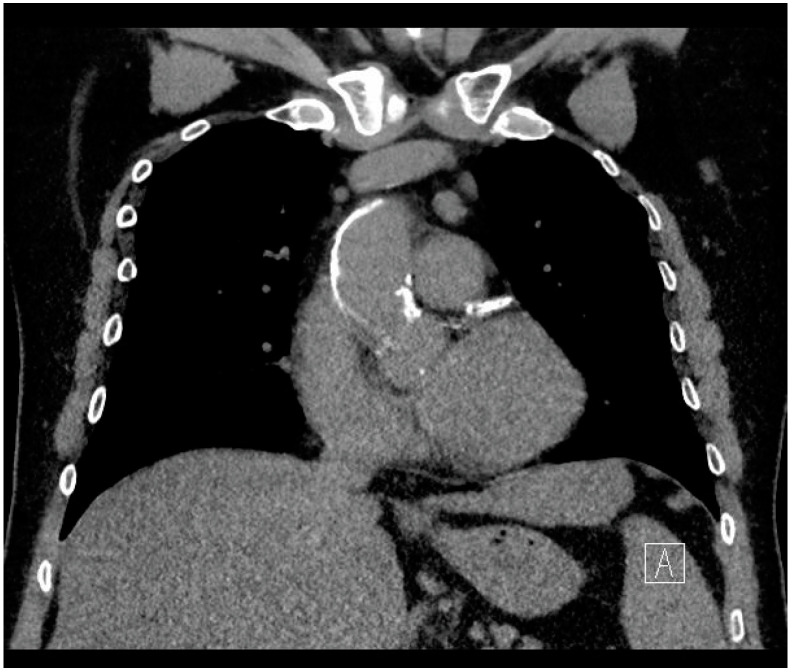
An example of massive calcifications detected by preoperative CT screening.
